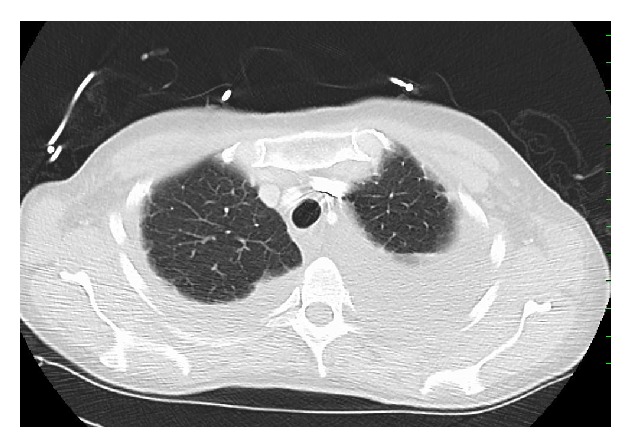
CT scan of the chest showing bilateral pleural effusions.
